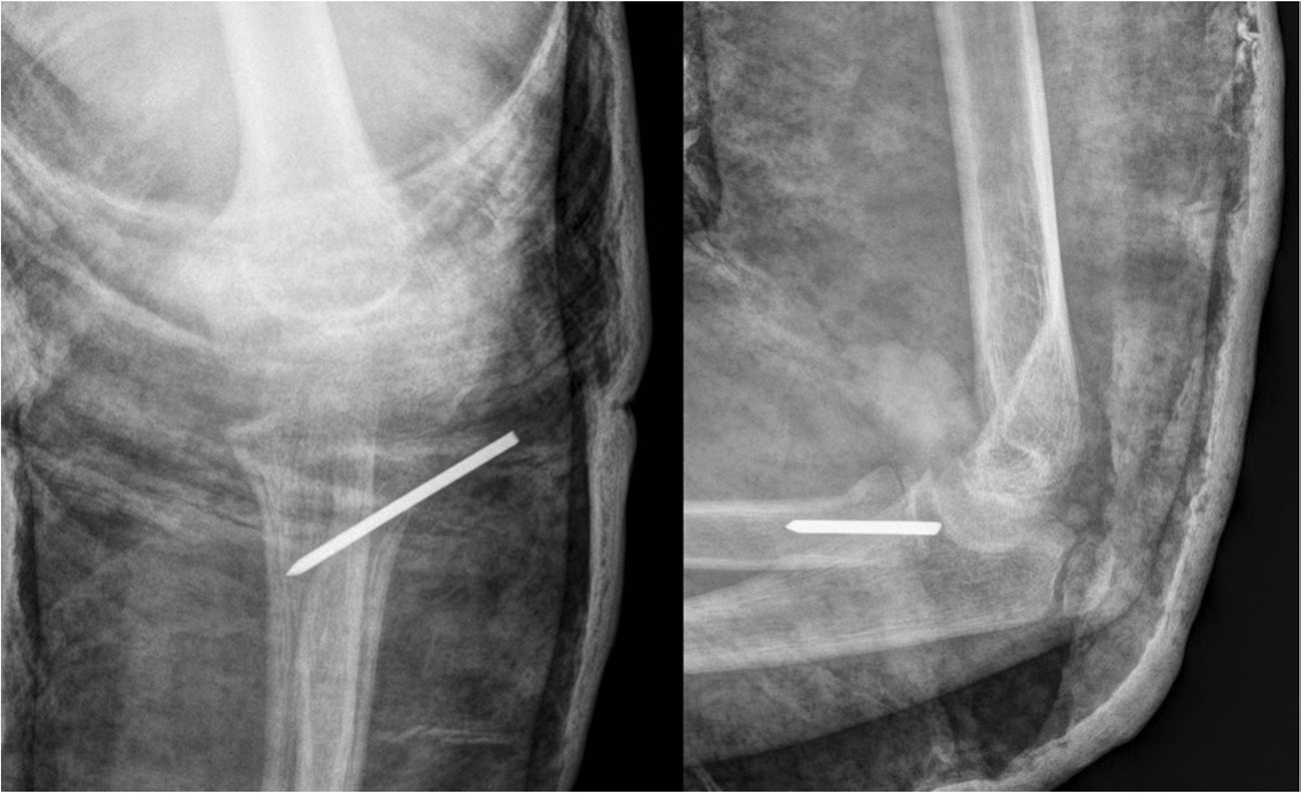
AP and L view radiograph of the elbow after surgical treatment by percutaneous leverage with Kirschner wire stabilization
